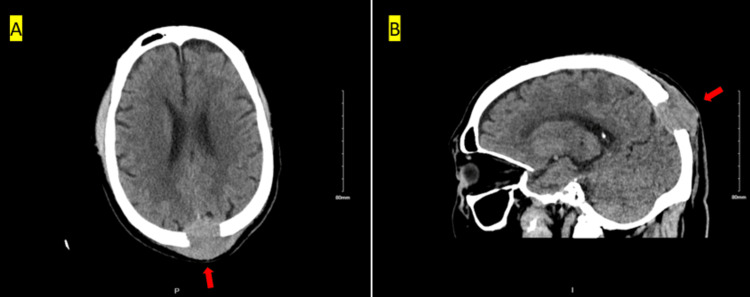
CT head without contrast 5.0 x 4.2 x 3.3 cm of soft tissue mass centered within the dorsal midline parietal-occipital bone with extension into the epidural space and invasion of the superior sagittal sinus. The mass slightly compresses the underlying parietal and occipital cortex without associated vasogenic edema.
